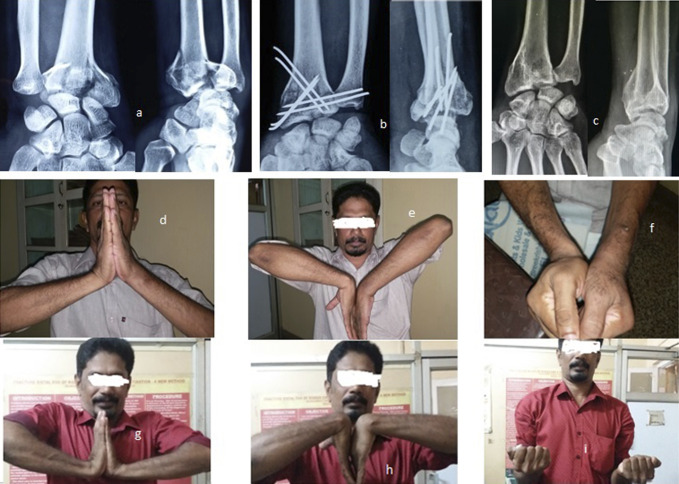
Photograph showing a 46-year-old man from day 1 to 1 year (left wrist). A, Pre-op radiograph. B, Post-op radiograph. C, radiograph at 1 year. D, Dorsiflexion at 6 weeks. E, Palmar flexion at 6 weeks. F, Grip at 6 weeks. G, Dorsiflexion at 1 year. H, Palmar flexion at 1 year. I, Grip and supination.
